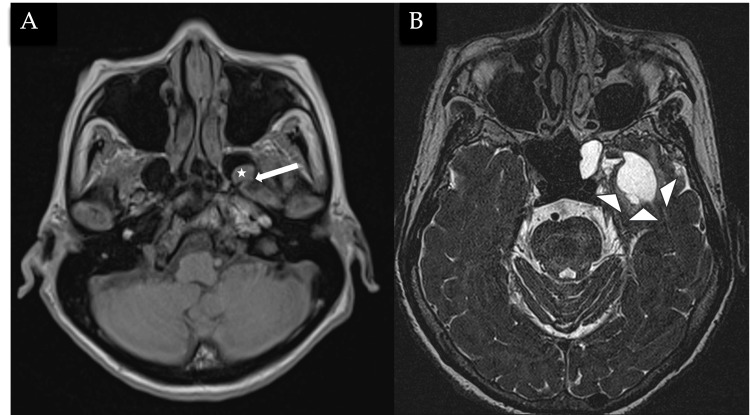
MRI T1 sequences in the axial (6A) showing encephalocele (white star) within the left sphenoid sinus, secondary to a defect in the left posterolateral sphenoid sinus wall (white box arrow). Axial T2 sequences (6B) showing cystic gliosis/encephalomalacia changes in the left temporal lobe (white arrowheads), probably sequelae of chronic herniation.
